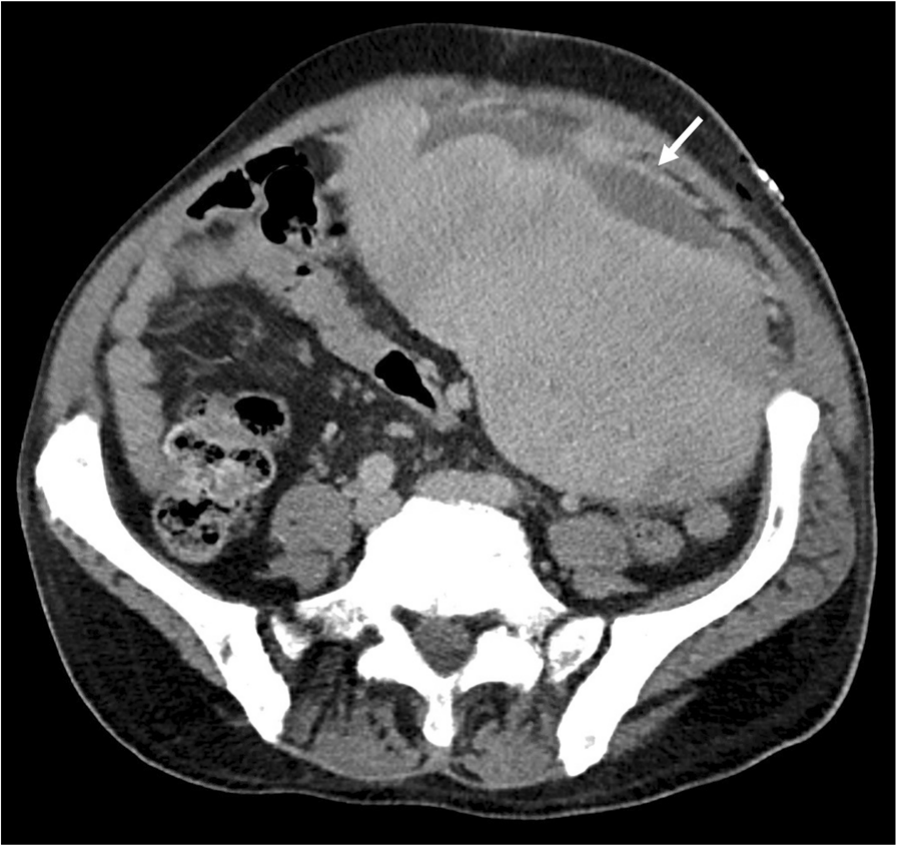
Axial CT of the abdomen demonstrates a chronic subcapsular splenic haematoma in a markedly enlarged spleen (white arrow). Severe organomegaly increases the risk of organ rupture with minor trauma or even spontaneously
